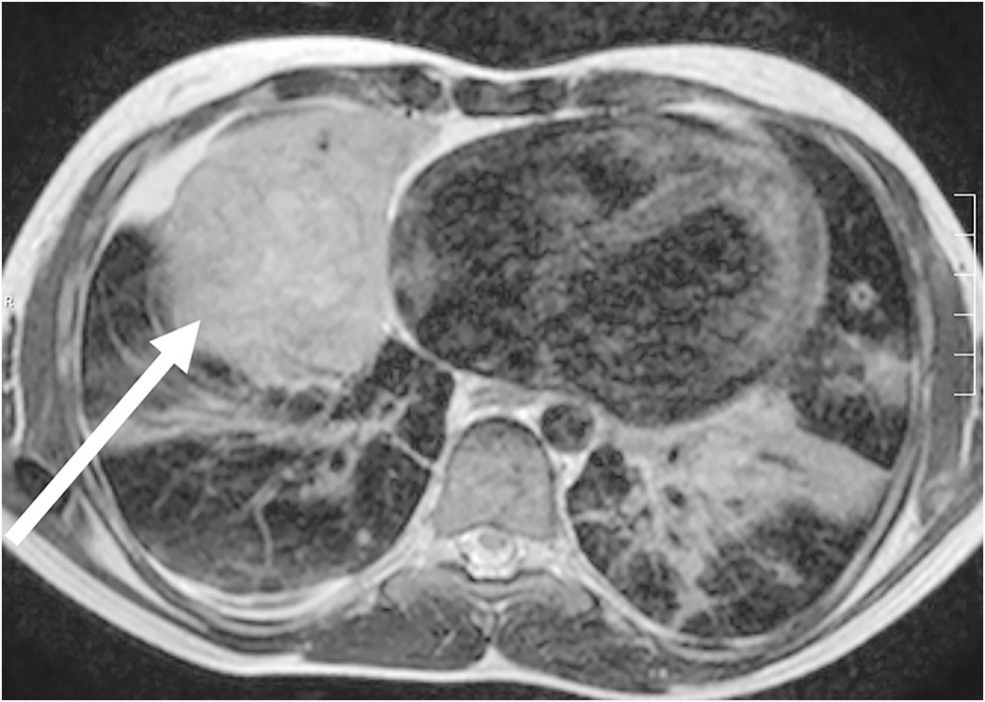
Lung MR in a 16-year-old girl with severe combined immunodeficiency. Axial T2-weighted turbo spin-echo image (MR parameters, Table 2) shows an inflammatory myofibroblastic tumor (*arrow*) in the right middle lobe
